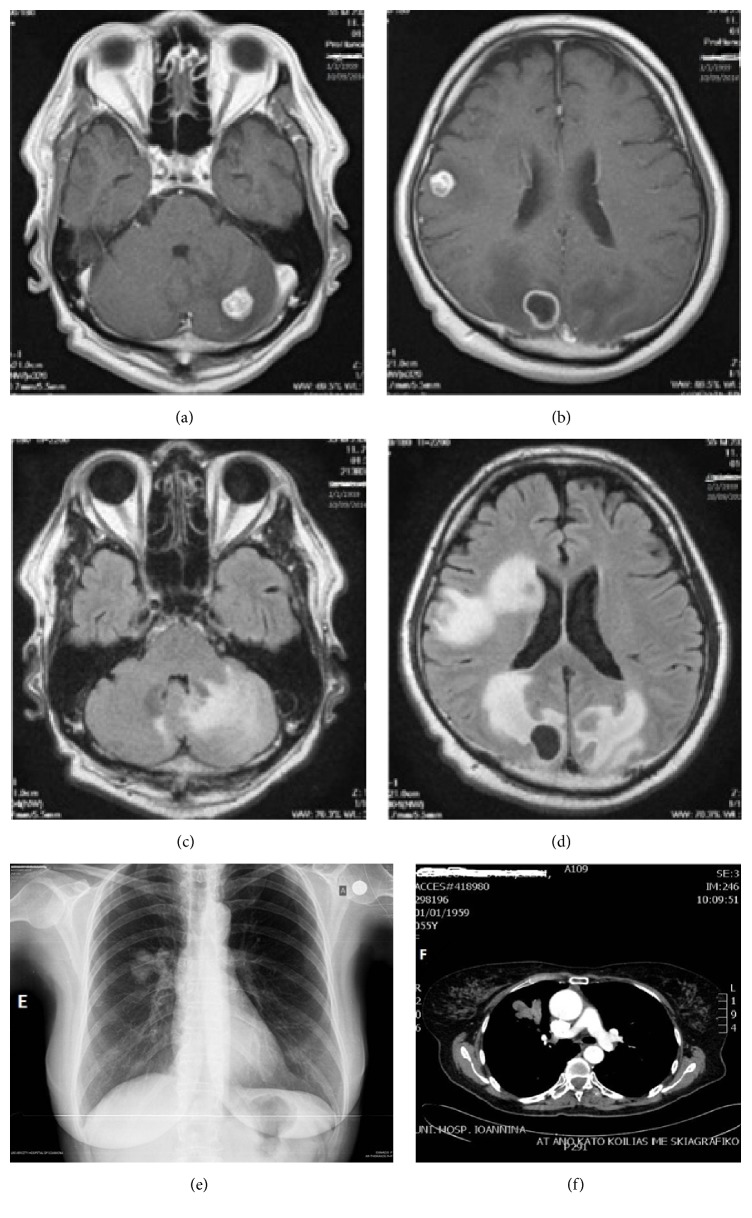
((a) and (b)) Postgadolinium T1-weighted MR images demonstrate multiple enhancing nodular lesions throughout both cerebral and cerebellar hemispheres, several of which have an unusual lamellated pattern of enhancement. ((c) and (d)) T2-weighted and FLAIR MR images with paired postgadolinium T1-weighted images demonstrate significant vasogenic edema associated with several of these lesions. (e) Chest X-ray showing tumor in the anterior segment of right upper lobe. (f) Chest CT showing tumor in the anterior segment of right upper lobe with ill-defined shape and right hilum lymph nodes.
